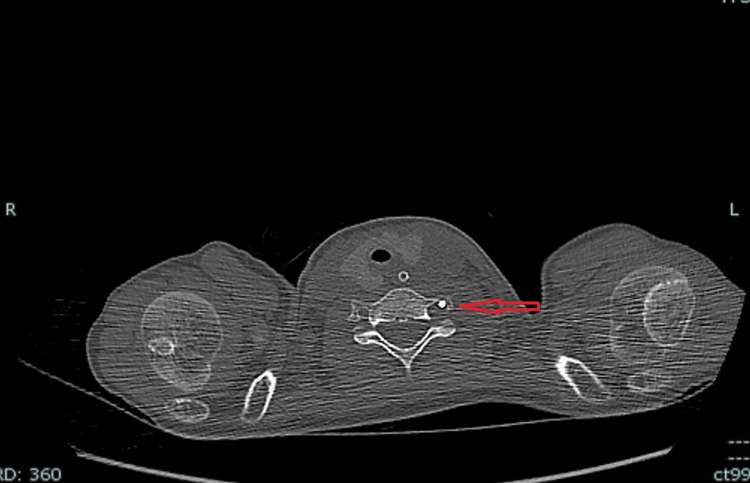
Computed tomography showing the central venous catheter (red arrow) passing through the left transverse foramen of C7.
